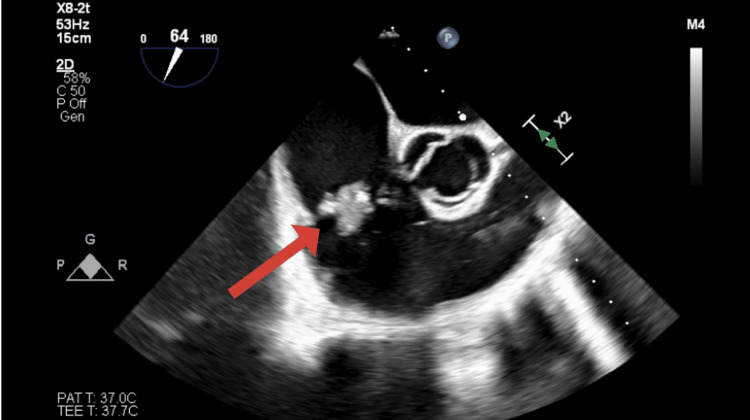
Transesophageal echocardiogram (TEE) of the tricuspid valve. Transesophageal echocardiogram (TEE) showing a large vegetation on the tricuspid valve, consistent with infective endocarditis in the setting of intravenous drug use (IVDU).
